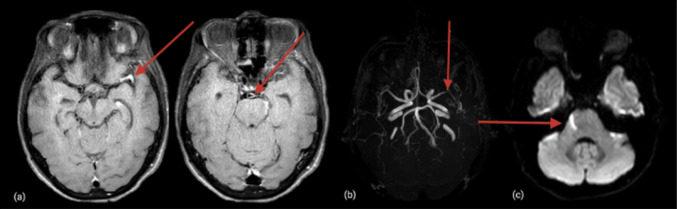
MRI of the cerebrum, case 4, 2024. See Tables 1, 2, 3 for case 4. a HRVWI-MRI with concentric enhancement of the BA, left SCA, left M1 and M2 branches of the MCA (red arrows). b MRI-TOF with stenosis of the left M1 and M2 branches of the MCA (red arrow). c subacute pontine infarction on the right side (red arrow). HRVWI-MRI (MRI High resolution vessel wall imaging), BA (basilar artery), SCA (superior cerebellar artery), MCA (middle cerebral artery), TOF (time of flight)
